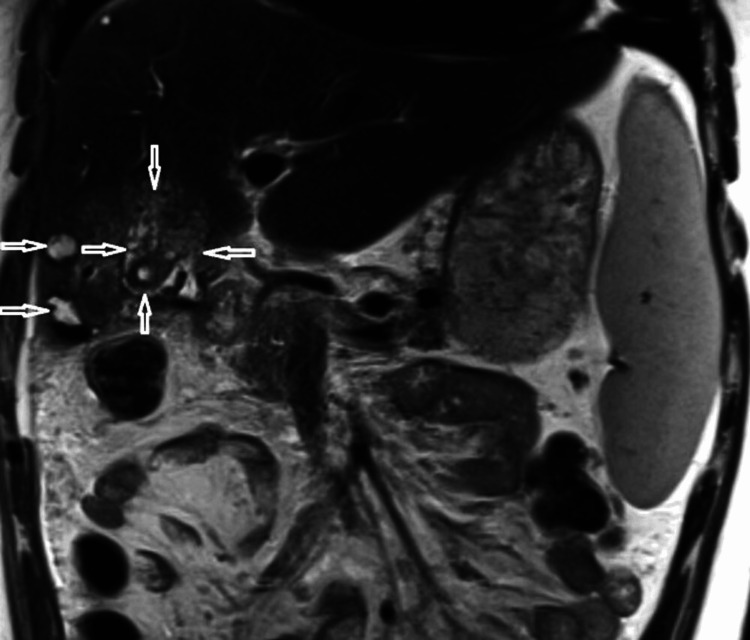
Selected coronal section of magnetic resonance imaging (T2) after stent and biopsy showing an enlargement of the T2 heterogeneous area on segment IV with multiple adjacent small abscesses (arrows)
